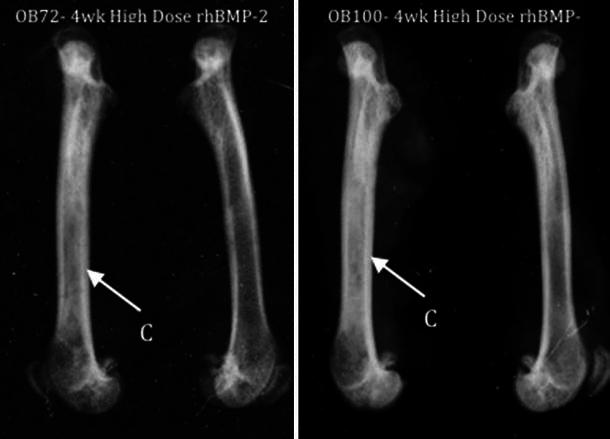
Magnified lateral radiographs of re-sected femora at 4 weeks. *C* Qualitative observation of cortical thickening in rhBMP-2 treated femurs. Note: treated (right) femurs appear on the *left* in both radiographic images
